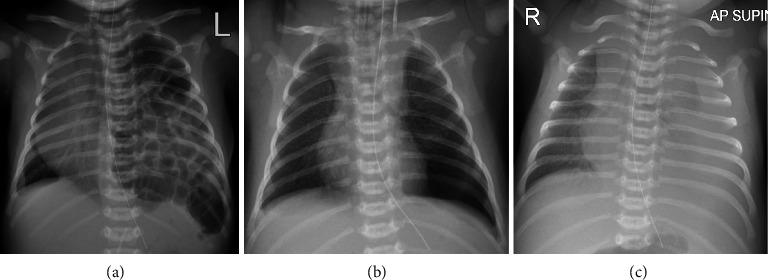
Chest radiographs for the subject. (a) Preoperative film with bowel loops in the left hemithorax. (b) Postoperative with normal appearance. (c) “Whiteout” of the left hemithorax at Day 10 of life caused by chylothorax.
